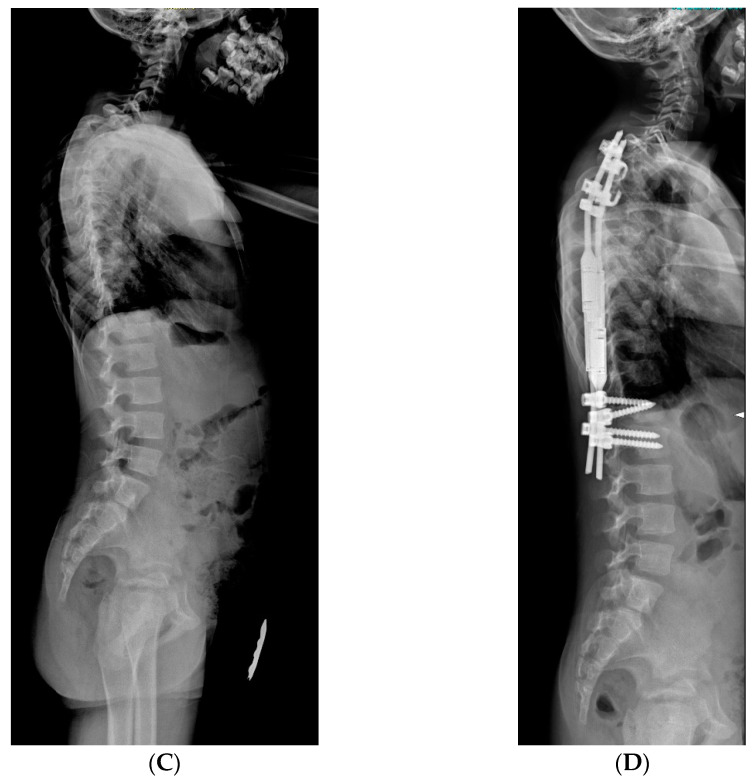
X-Rays of early onset scoliosis (EOS) corrected with magnetically controlled growing rods (MCGR): (A) pre-operative antero-posterior X-ray view; (B) post-operative antero-posterior X-ray view; (C) pre-operative lateral X-ray view; (D) post-operative lateral X-ray view.
